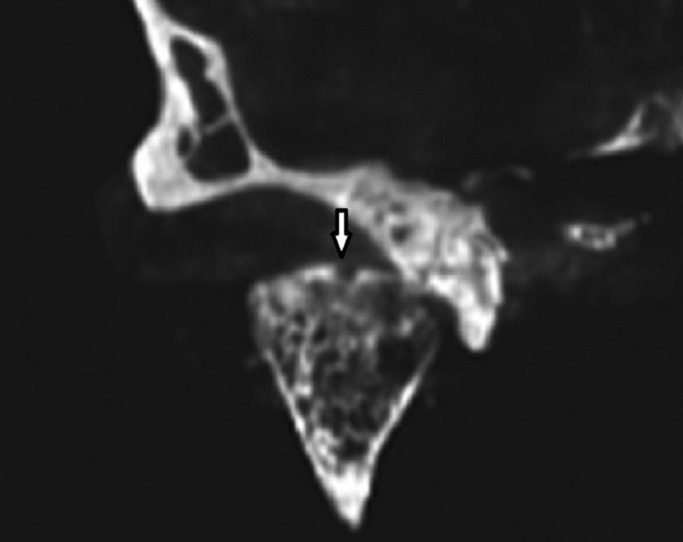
CBCT image shows coronal view of an erosive lesion on condylar head.
